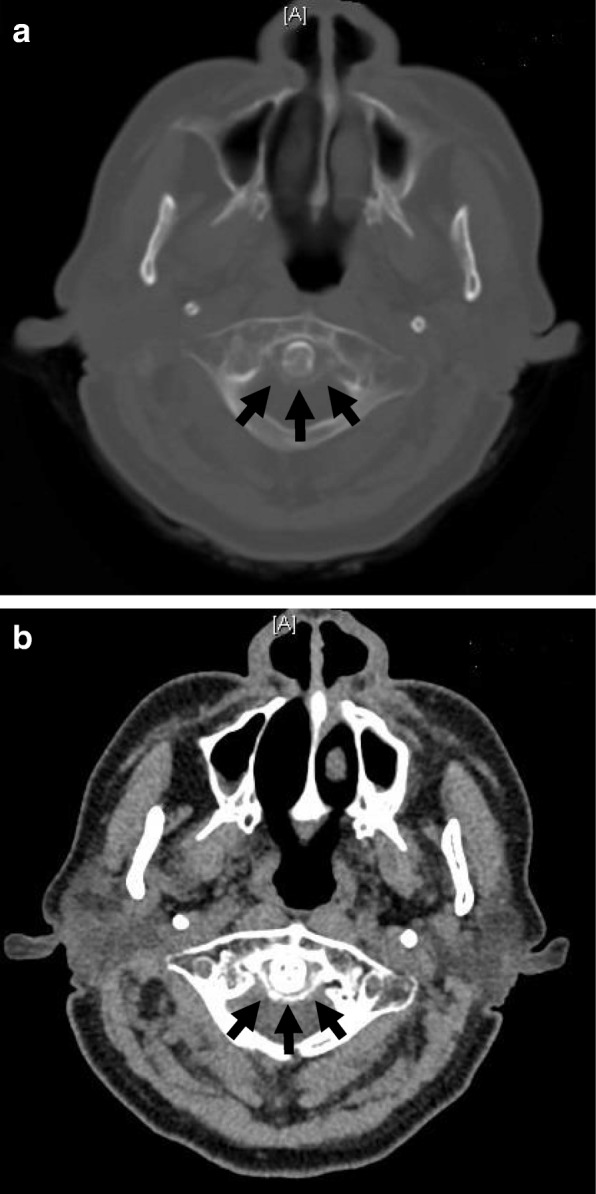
Contrast computed tomography image of the neck on day 10 of hospitalization. a Axial image in bone windows (width = 2000, center = 400); although there is evidence of calcification around the odontoid process of the second cervical vertebra, it is pale and fine (*arrows*). b Axial image in soft tissue windows (width = 250, center = 30); calcification around the odontoid process of the second cervical vertebra is clearly visible (*arrows*)
